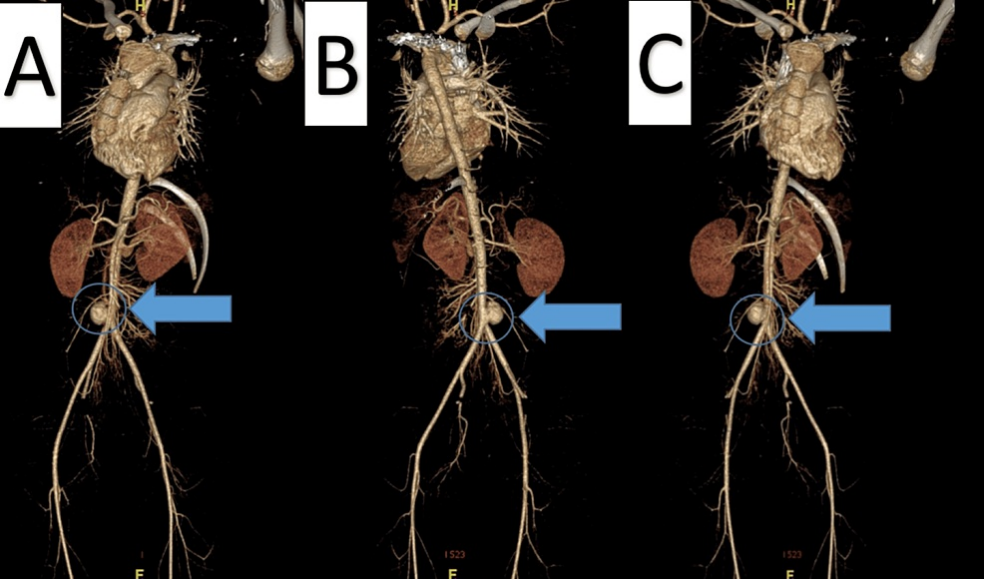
Chest and Abdominal Computed Tomography Angiogram A: Reformate angiography demonstrating descending aorta shows aortic aneurysm (blue arrow); B: It arises from the right side of the aorta just before its bifurcation to the iliac arteries (blue arrow); C: The aortic aneurysm is anterior and shows a wide neck, measuring 1.4 cm (blue arrow).
